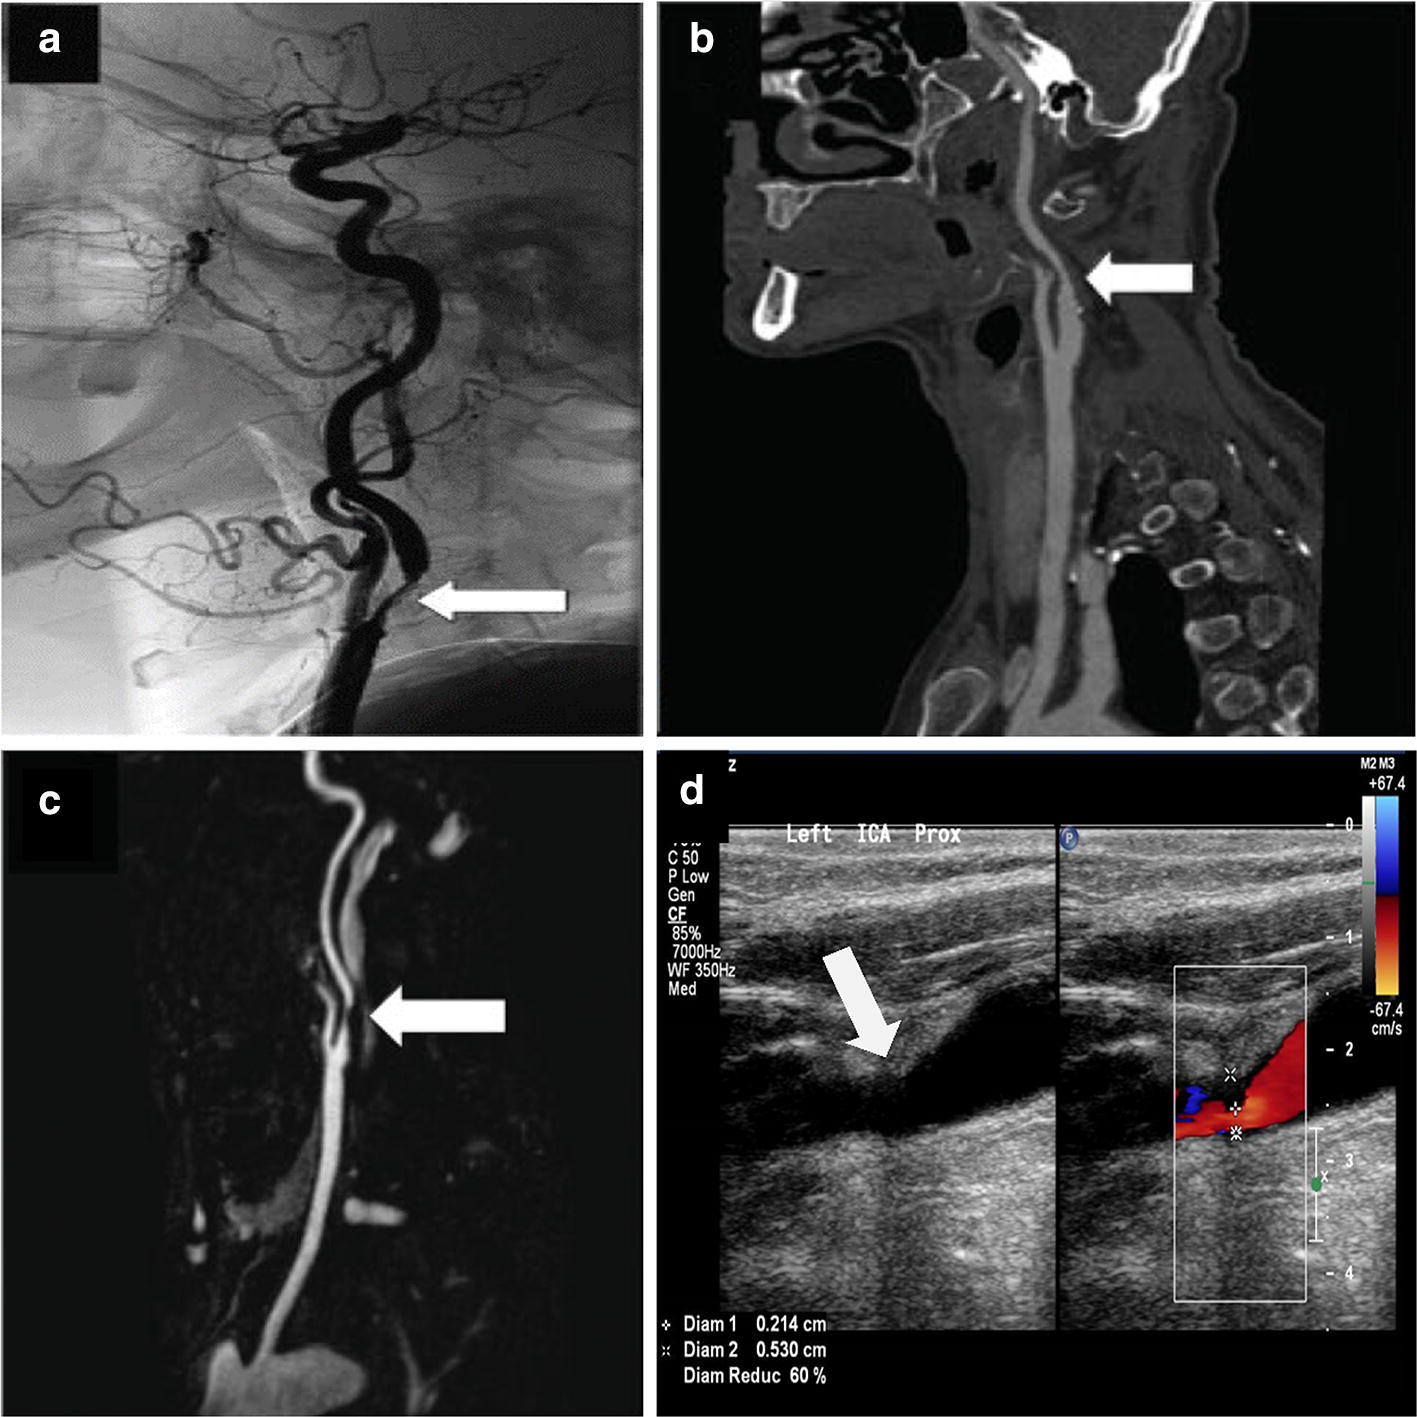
ICA stenosis visualization with a white marker arrow in a DSA image [75], b CTA image [75], c MRA image [75], and d DUS image (credits: National Heart Center Singapore)
